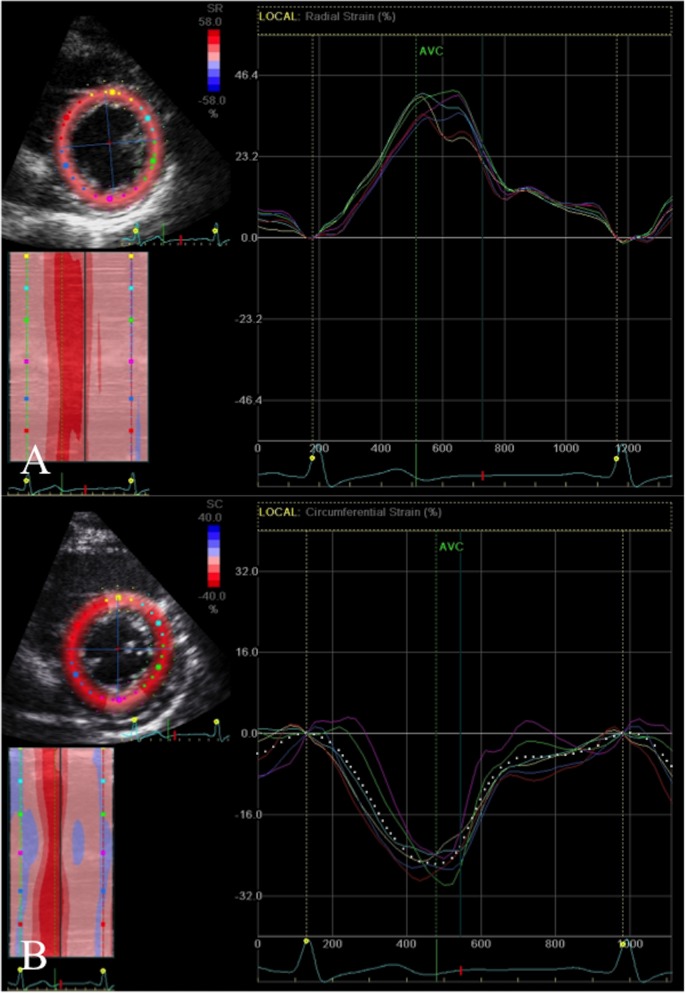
Radial and circumferential strain. A. Radial strain (peak systolic positive deflection) and B. Circumferential strain (peak systolic negative deflection) from the parasternal short axis view at the level of the papillary muscles (septal, anteroseptal, anterior, lateral, posterior, inferior segments). X-axis = Time (s); Y-axis = Strain (%).
